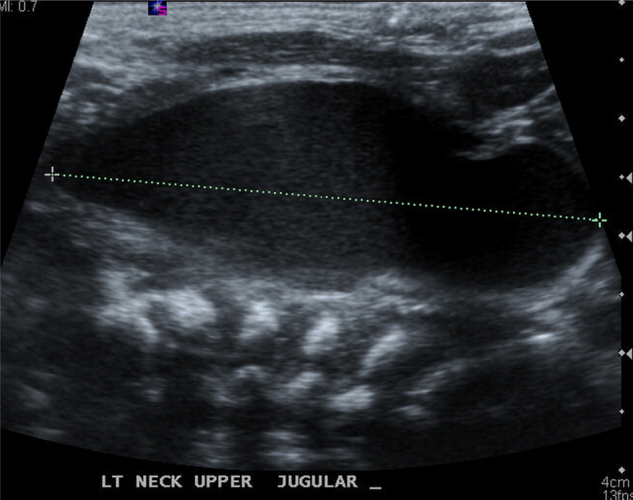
On ultrasound scan the neonatal huge neck mass (4.0 cm × 2.3 cm × 1.5 cm) was described as cystic mass with anechoic area.
